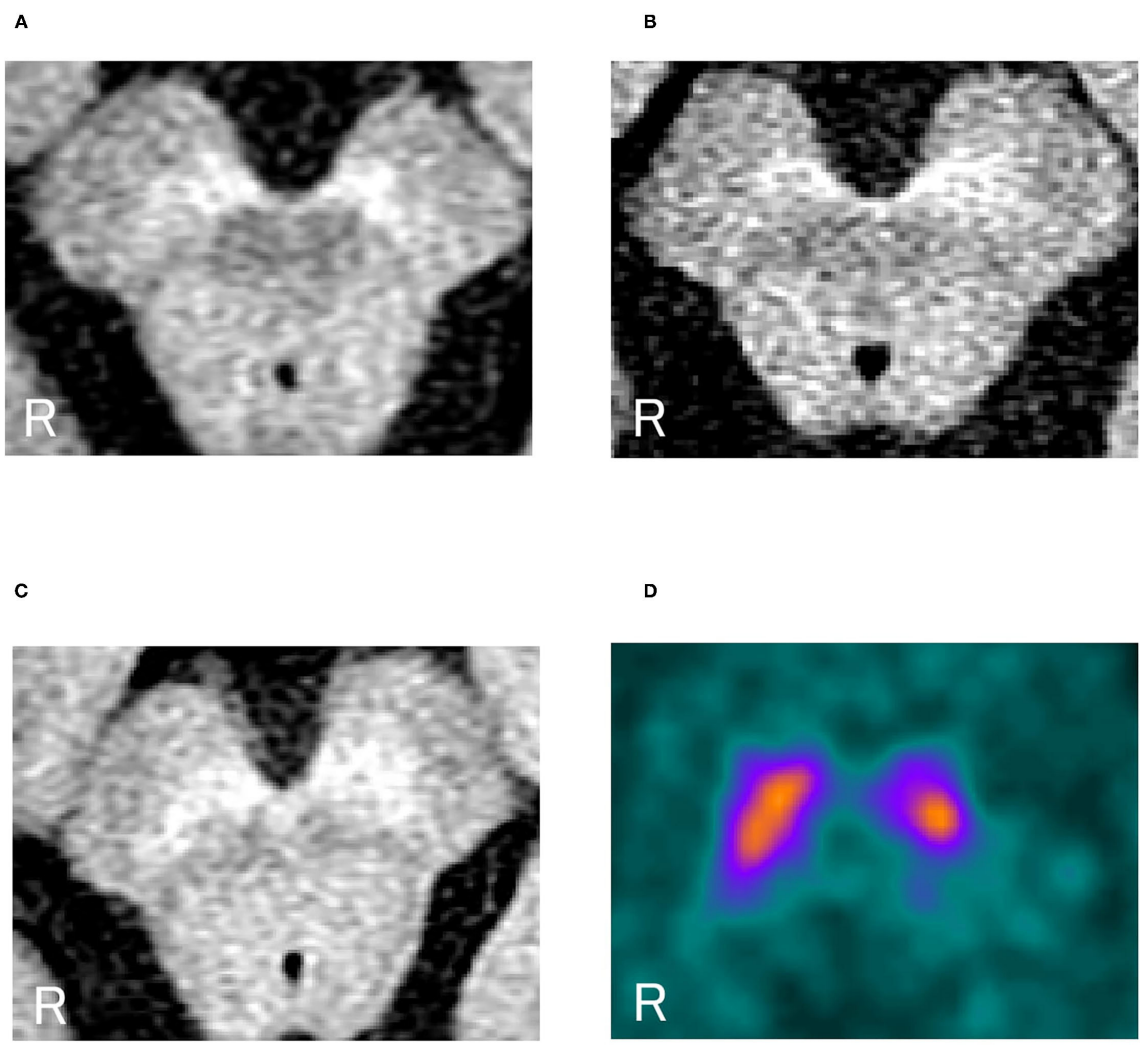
(A) Neuromelanin-sensitive magnetic resonance imaging in a representative dystonia case (a patient with DYT-*SGCE*). (B) NM-MRI in a representative PD case. (C) NM-MRI in a representative control case. (D) DAT SPECT in a representative case of a female patient with dystonia (DYT-*SGCE*). Her cSBR was 4.79/3.33. Her age-estimated cSBR was 8.40. The 95% lower limit of prediction intervals on the cSBR for 50–59 years was 5.95. NM-MRI, neuromelanin magnetic resonance imaging; DYT-*SGCE*, dystonia*-epsilon sarcoglycan* gene; PD, Parkinson's disease; DAT SPECT, dopamine transporter single-photon emission contrast tomography; cSBR, calibrated specific binding ratio.
